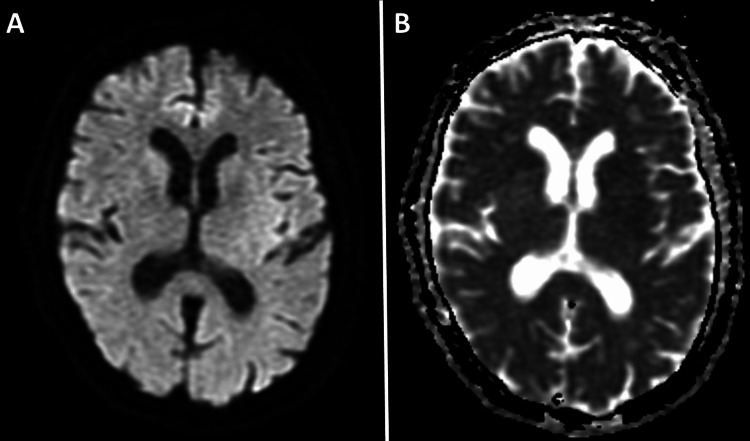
(A) Axial diffusion-weighted MRI of the brain (repetition time msec/echo time msec, 8500/100; flip angle, 90°; b value = 1000 sec/mm2) and (B) corresponding axial apparent diffusion coefficient maps (8500/100; flip angle, 90°; b value = 1000 sec/mm2) demonstrate the absence of restricted diffusion.
